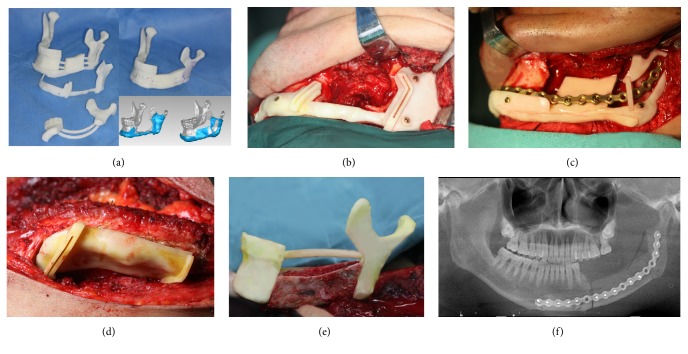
Outlines for the CGT (complicated guiding templates). (a) Overview of the complicated cutting guide, detachable reconstructive template, and in-site reshaping guide. (b) Application of the complicated cutting guide on the surface of mandible. (c) Application of the detachable reconstructive template that integrated with prebending titanium plate to restore the bony parts position after mandibulectomy. (d) Application of the cutting guide in iliac area. (e) Application of the in-site reshaping guide. (f) Postoperative panoramic radiograph showed the defect was reconstructed with iliac bone precisely.
